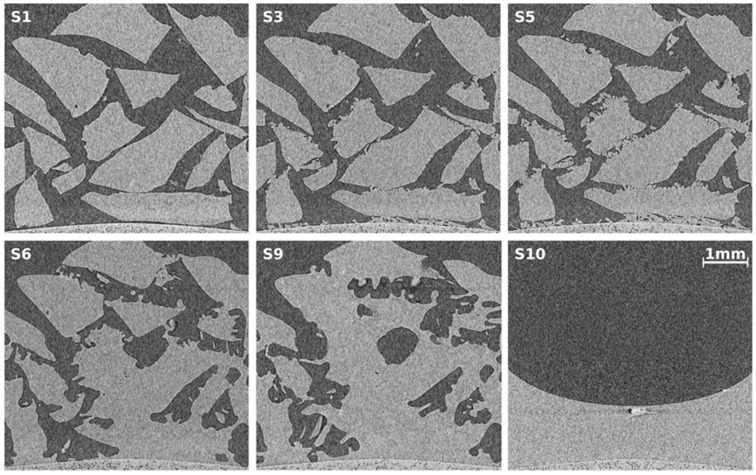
Tomographic images obtained from CT scans (numbered S1, S3, S5, S6, S9, and S10) of the ice sample at different points in time. Each slice represents the exact same position in space. In S1–S5 the sample temperature is 243 K, while in S6–S9 it is 270 K. S10 shows the meniscus of the molten sample. The bright points in slice S10 stem from tiny metallic particles that produced metal artifacts which had to be corrected manually.
